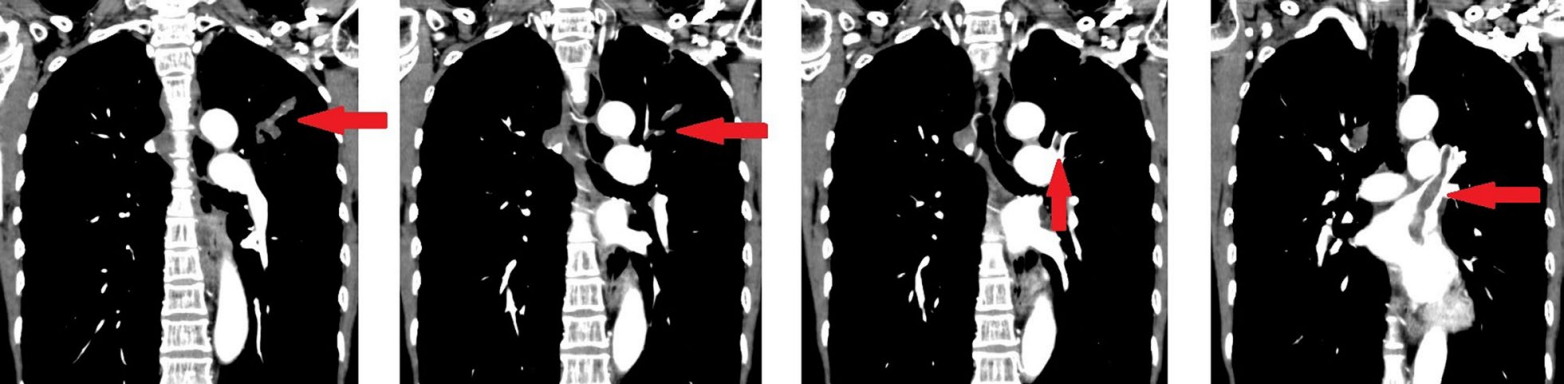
Contrast computed tomography showing enlarged lung metastases and that disease in the left-upper lobe has infiltrated the left superior pulmonary vein and left atrium
